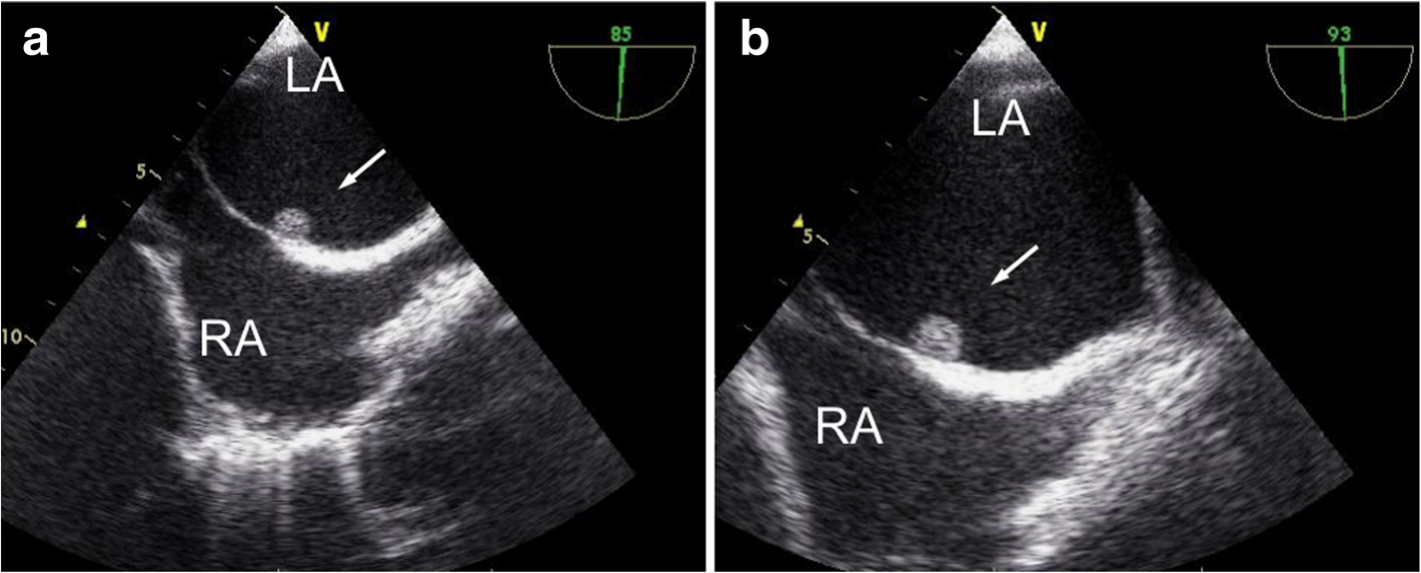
a, b Bicaval transesophageal echocardiograms, showing an acaulescent immobile mass in the left atrium arising from the atrial septum (*arrow*). Abbreviations: *LA* left atrium, *RA* right atrium
